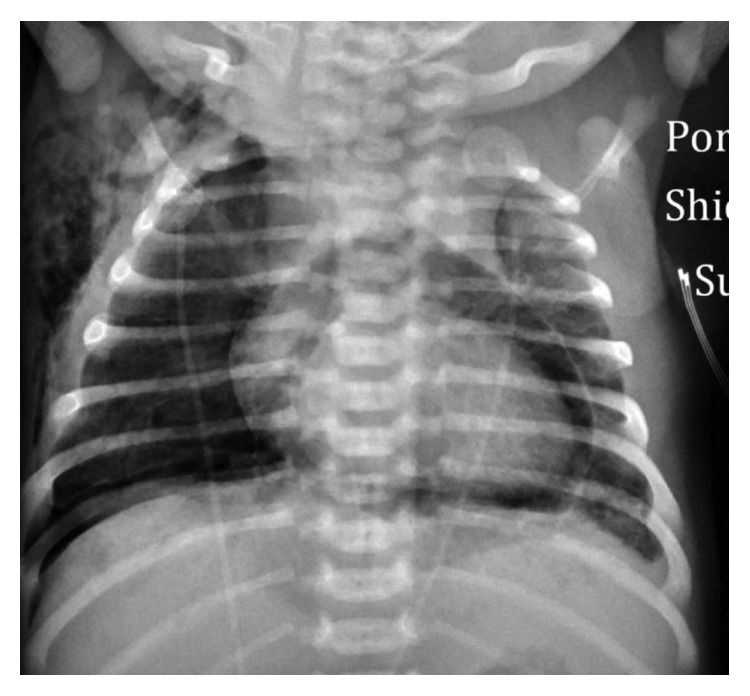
Anteroposterior chest radiograph showing pneumothorax on the right side, pneumopericardium, and subcutaneous emphysema over right axillary region.
